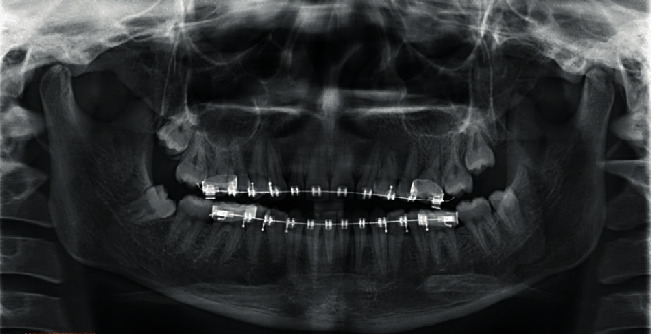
Orthopantomogram at the end of treatment.
